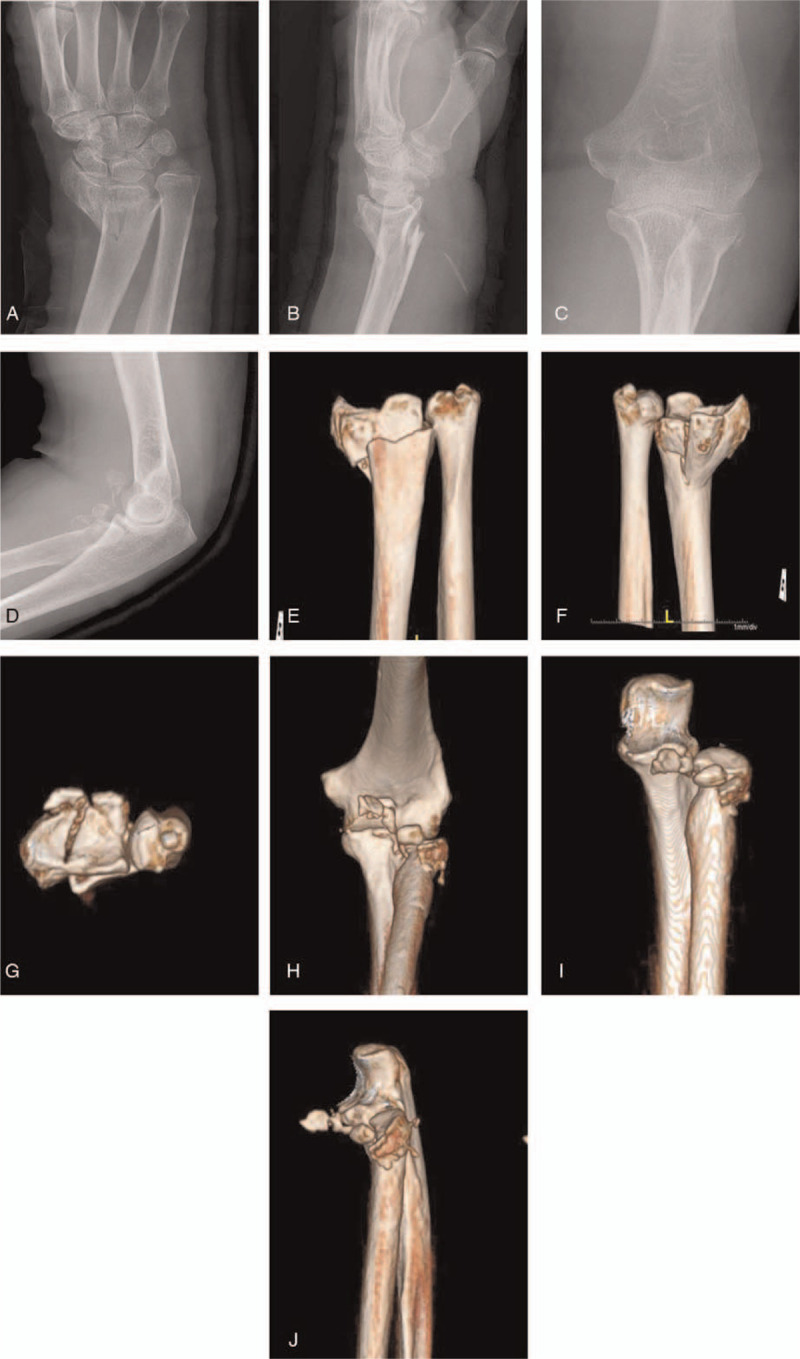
Preoperative plain radiographs of case 1. Anteroposterior and lateral X-ray images of the wrist (A, B) and the elbow (C, D) and CT images of the wrist (E-G) and the elbow (H-J) show an AO C3 distal radius fracture, a Mason type III radial head fracture, and Regan-Morrey type I coronoid process fractures.
